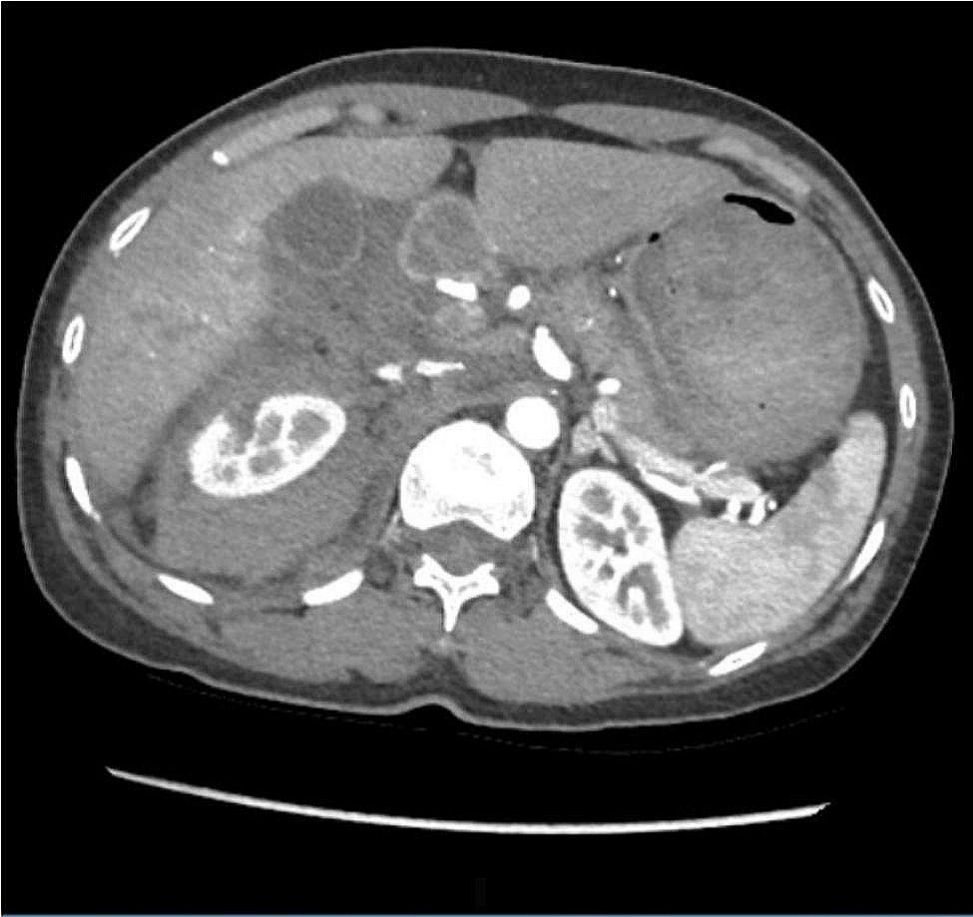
Abdominal CT (portal venous phase) imaging demonstrating high grade right kidney laceration with haemoperitoneum and liver laceration
